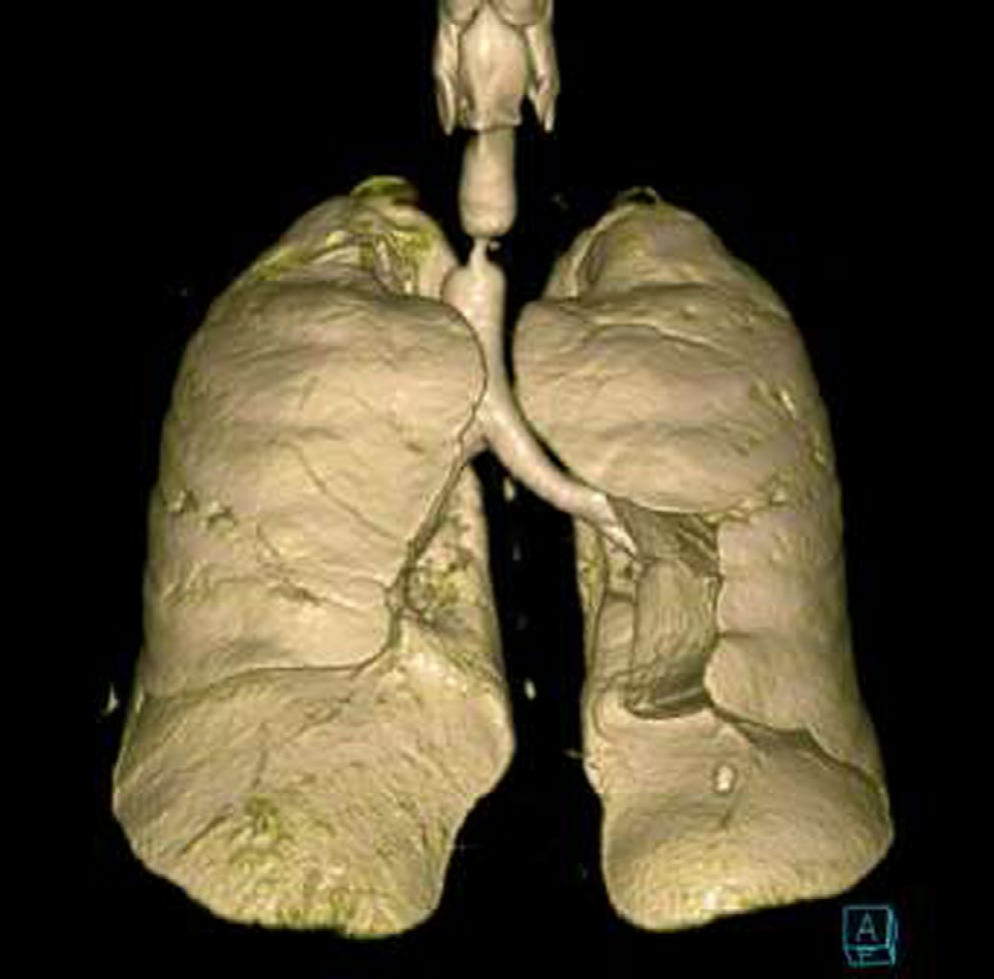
3D reconstruction of CT‐Chest showing the level and extent of the tracheal stenosis (anterior view)
